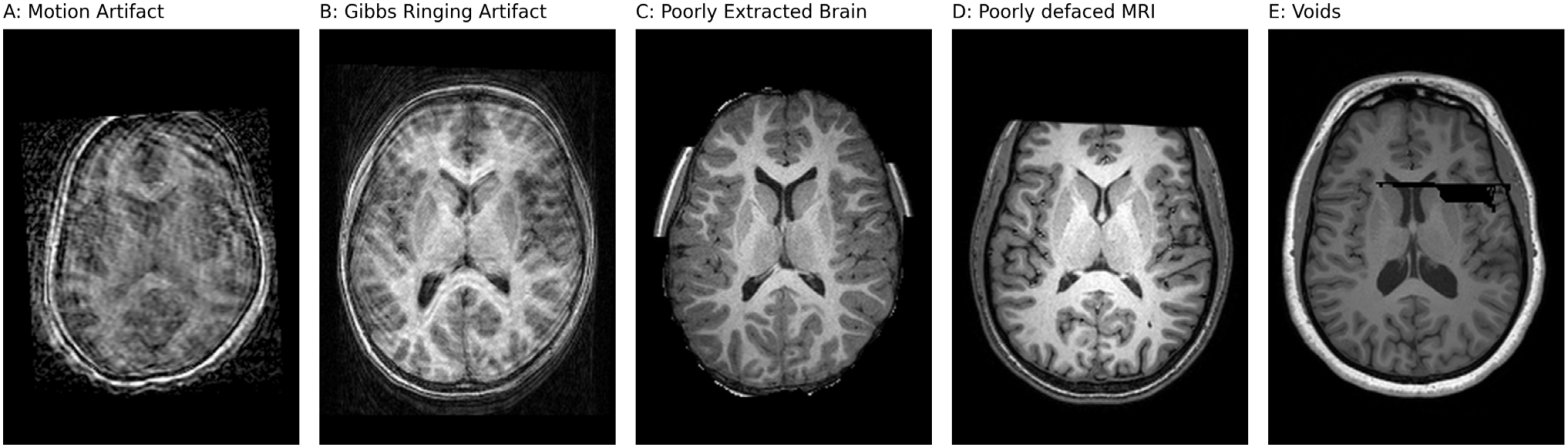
Examples of MRI scans excluded during visual quality inspection: (A) motion artifacts, (B) Gibbs ringing artifact (truncation artifact), (C) poorly extracted brain regions, (D) inadequate defacing with cropped brain regions, and (E) missing or void pixels.
